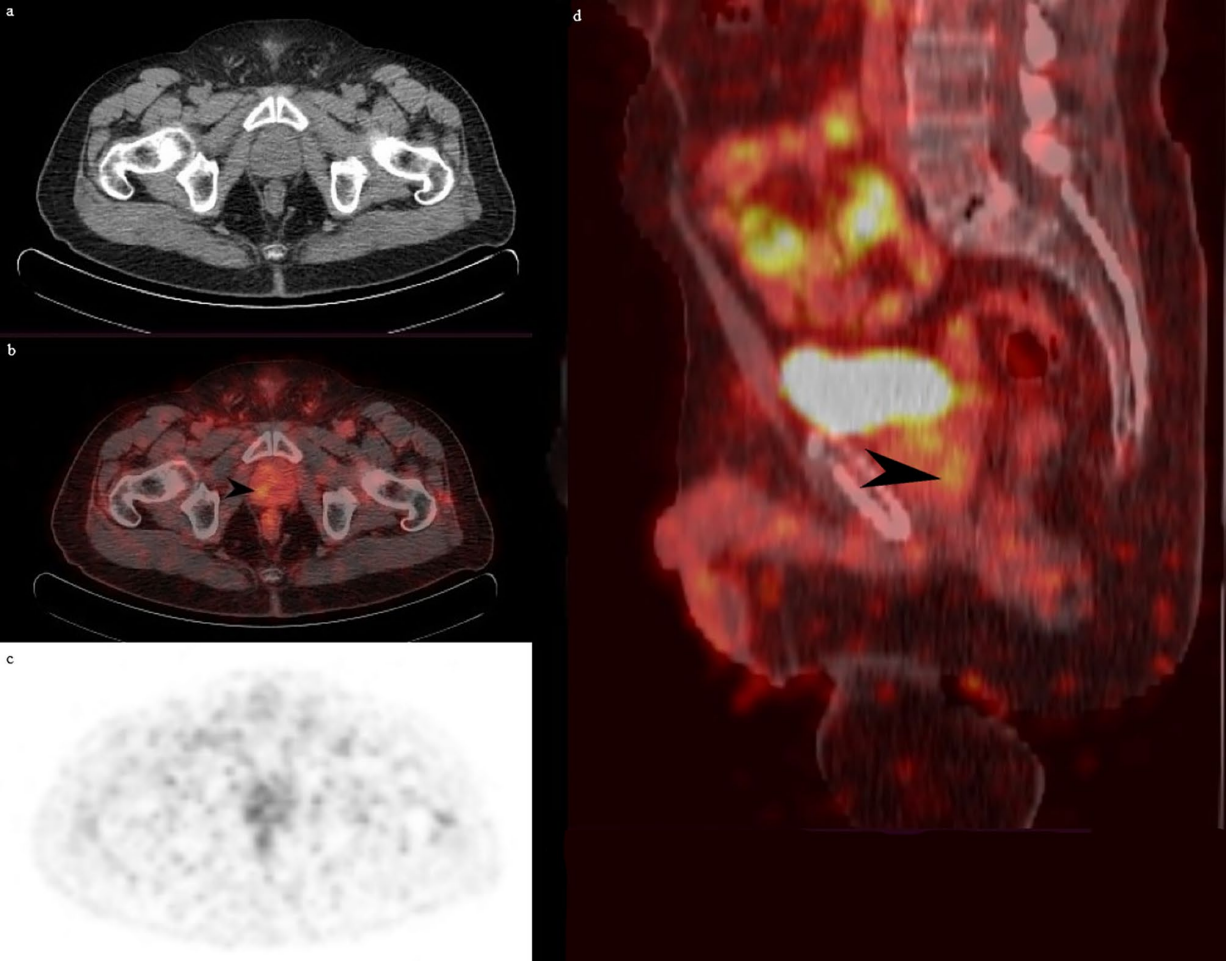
A 71-year-old patient on ADT and status post EBRT 60 Gray in 20 fractions. PSA at time of acquisition = 9.5 ng/mL. Axial CT (a) image of the prostate bed is shown. Fused PET/CT (b) shows focus of increased radiotracer uptake in the right peripheral zone of the prostate gland at mid-gland (black arrow) in keeping with disease recurrence. PET image is shown (c). Sagittal fused PET/CT (d) shows the described lesion (black arrow).
